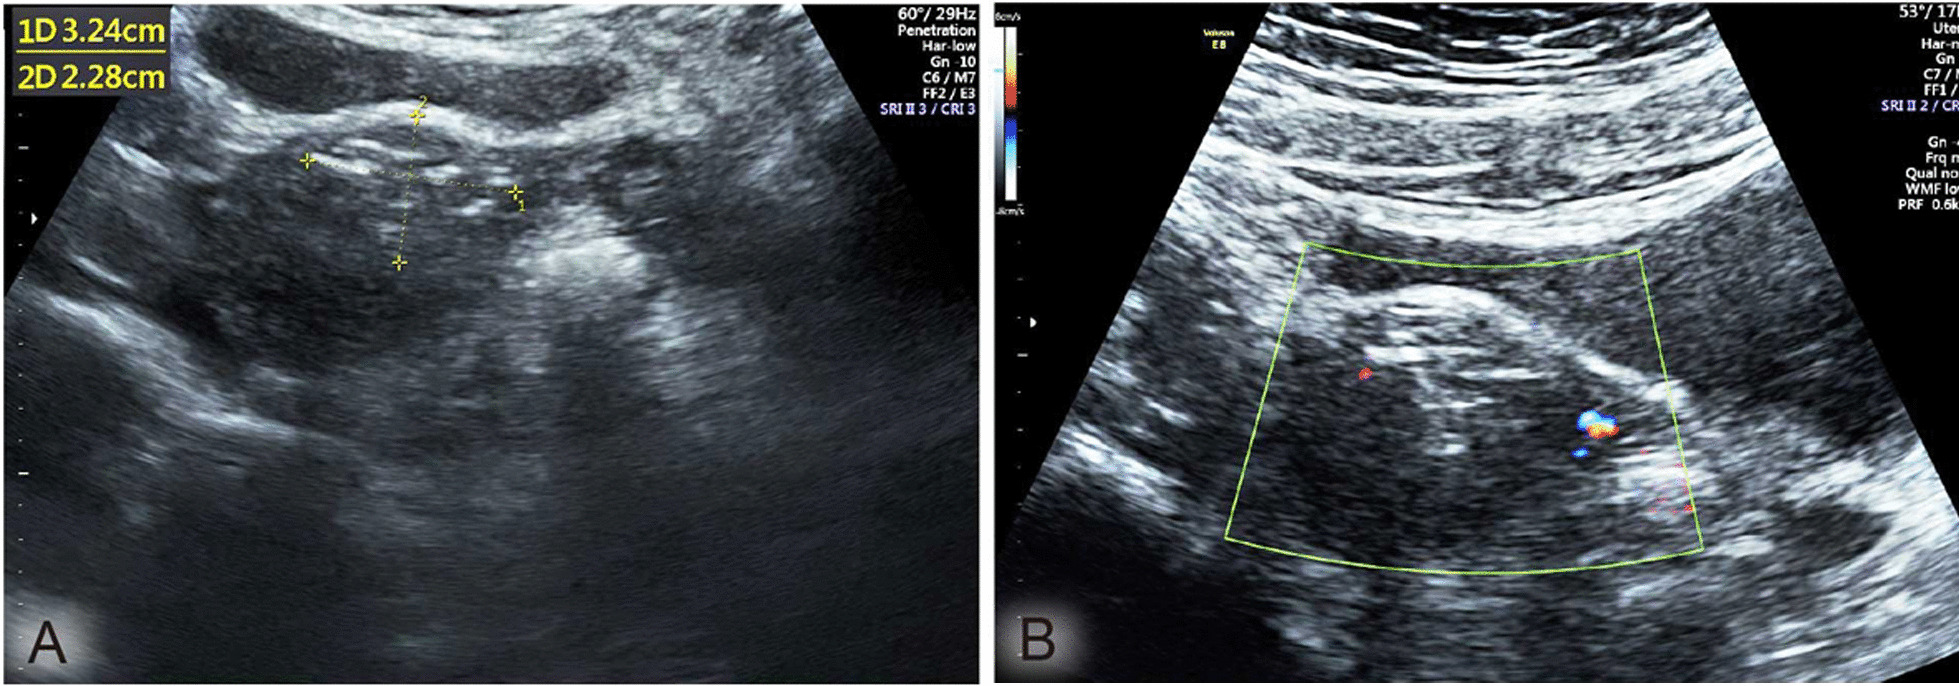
Sonography one month after the surgery: the myomectomy scar showed an ill-defined heterogeneous myometrial texture (A) with a slightly higher vascular signal compared with the surrounding myometrium (B). The maximum diameter line of the scar was measured to calculate the SRI
